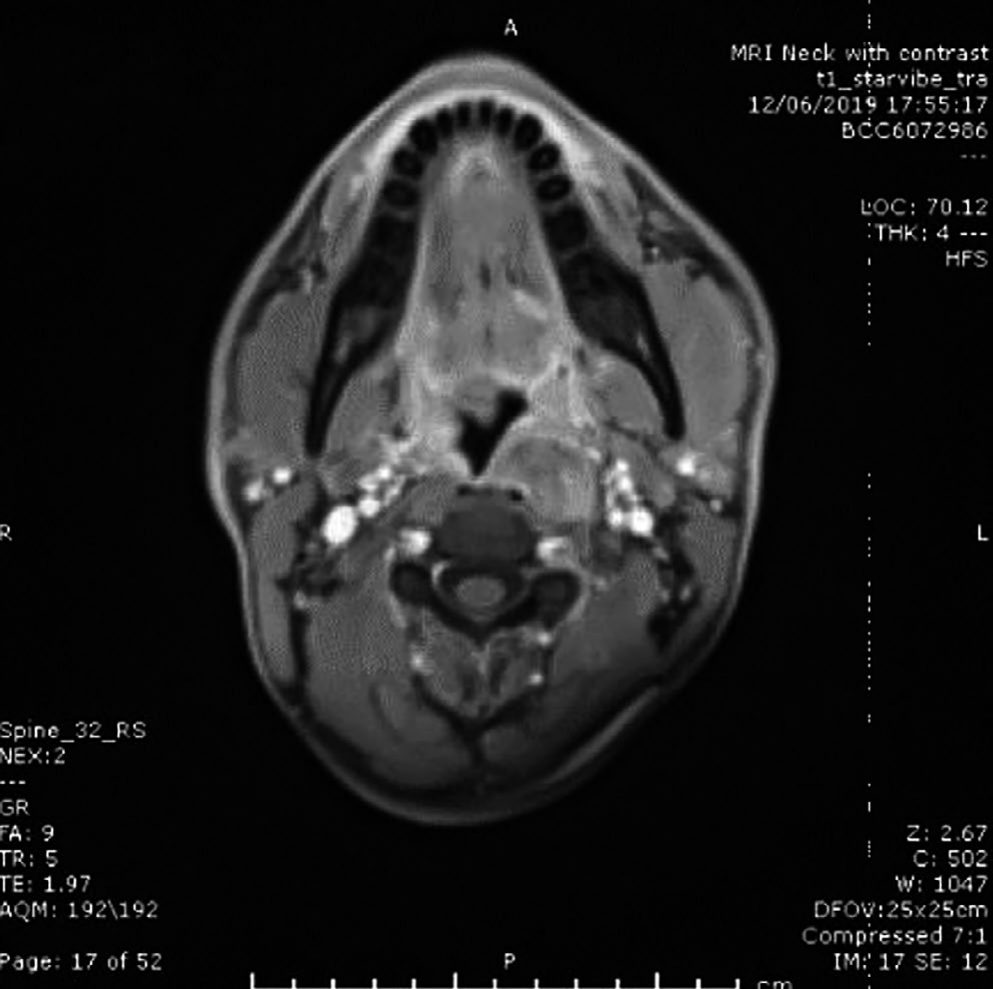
Sagittal T1 MRI section showing retropharyngeal soft tissue lesion (white arrow)
